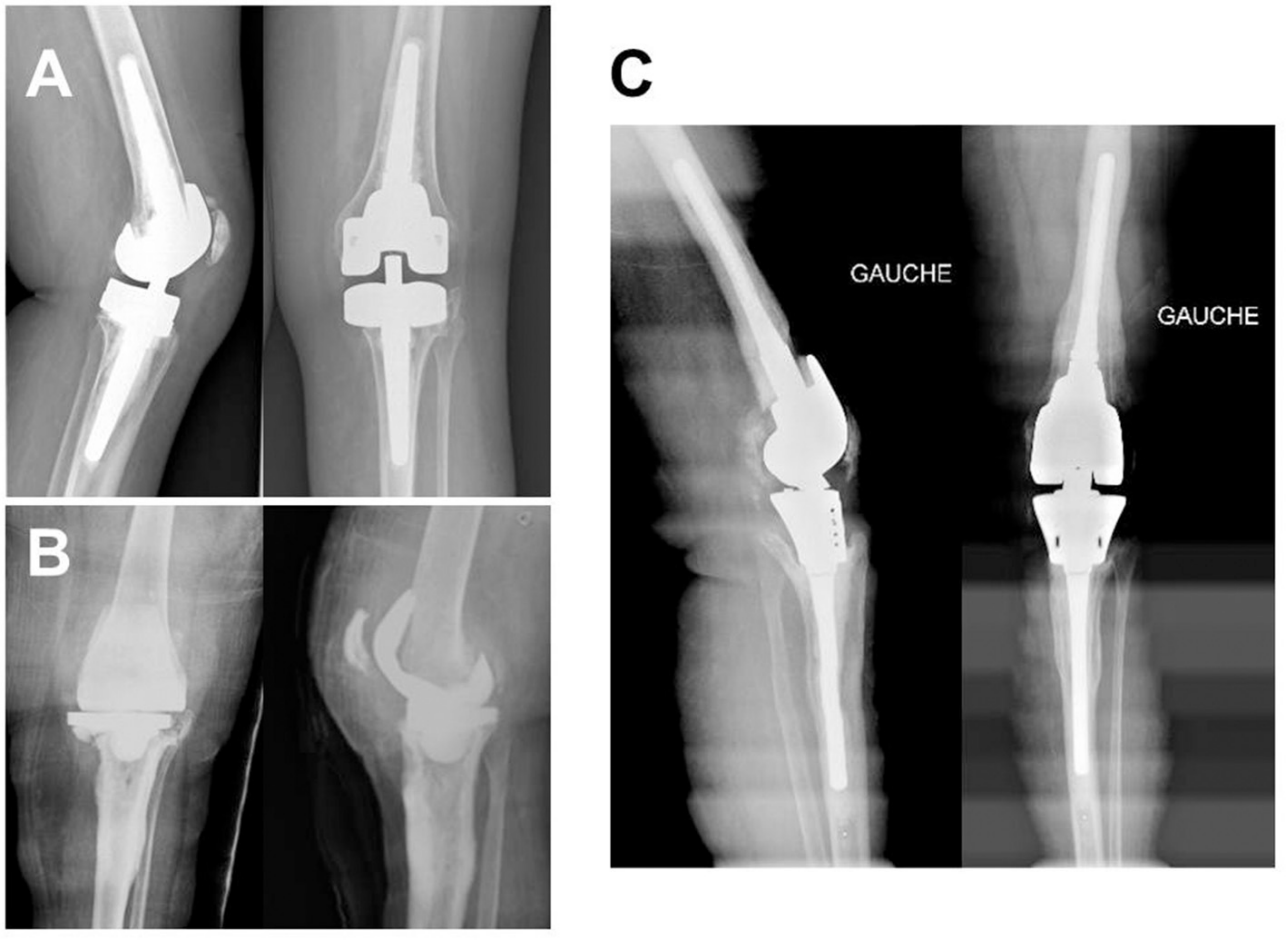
Typical x-ray from a patient with total knee arthroplasty (TKA) infection treated with a two-stage approach with reimplantation of a cemented hinged TKA: An 80-year-old female patient with a history of *Staphylococcus caprae* TKA infection (A) from whom explantation was performed (B, a gentamicin-cement spacer was used to fill the gap) and for whom reimplantation of a gentamicin-cemented hinged prosthesis because of important femoral and tibial bone loss AORI III (C). The outcome was favorable at 3 years of follow-up.
